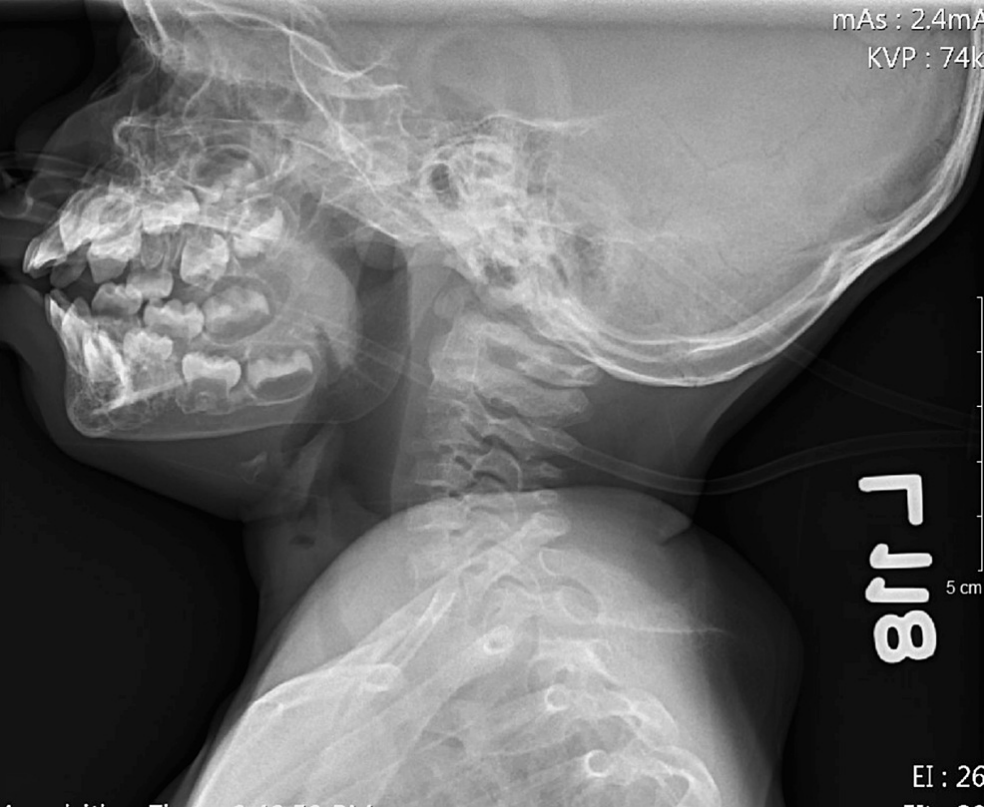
Lateral radiograph showing recurrent subglottic stenosis and diffuse tracheal narrowing on second pediatric intensive care unit (PICU) admission (one day after discharge from initial PICU)
